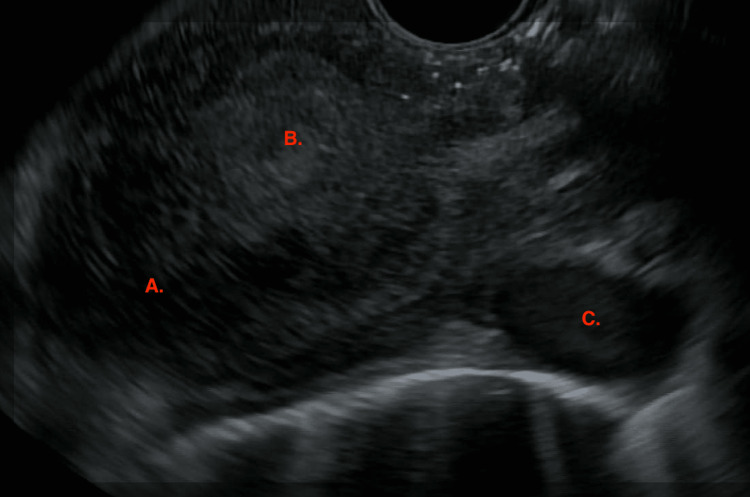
Endoscopic Ultrasound: partially circumferential mass involving the mucosa. A: Esophageal lumen B: Mass C: Malignant lymph node
